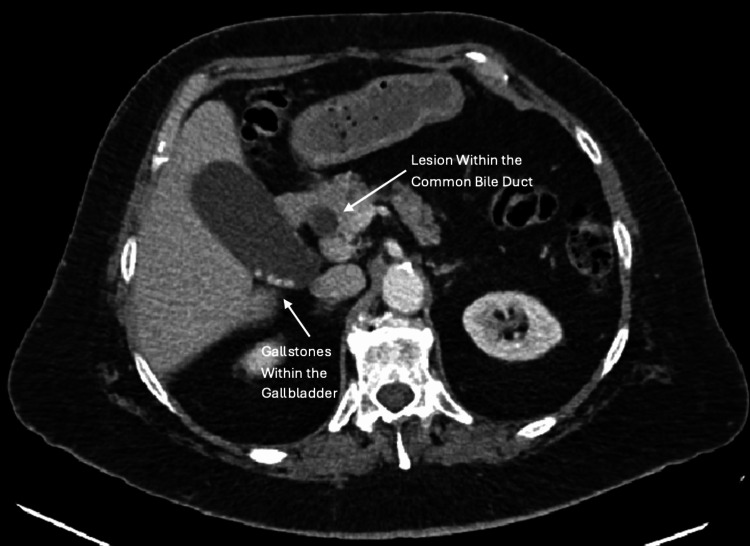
Computed tomography of the abdomen and pelvis. Gallstones are noted within the gallbladder, with a lesion identified within the common bile duct.
